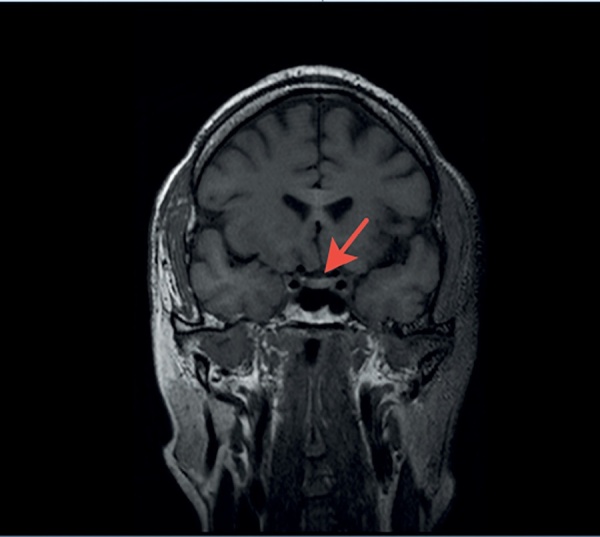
Рисунок 1. МР-картина хиазмально-селлярной области пациента. Фронтальный срез.Figure 1. MRI of the patient's chiasmal-sellar area. Front cut.
